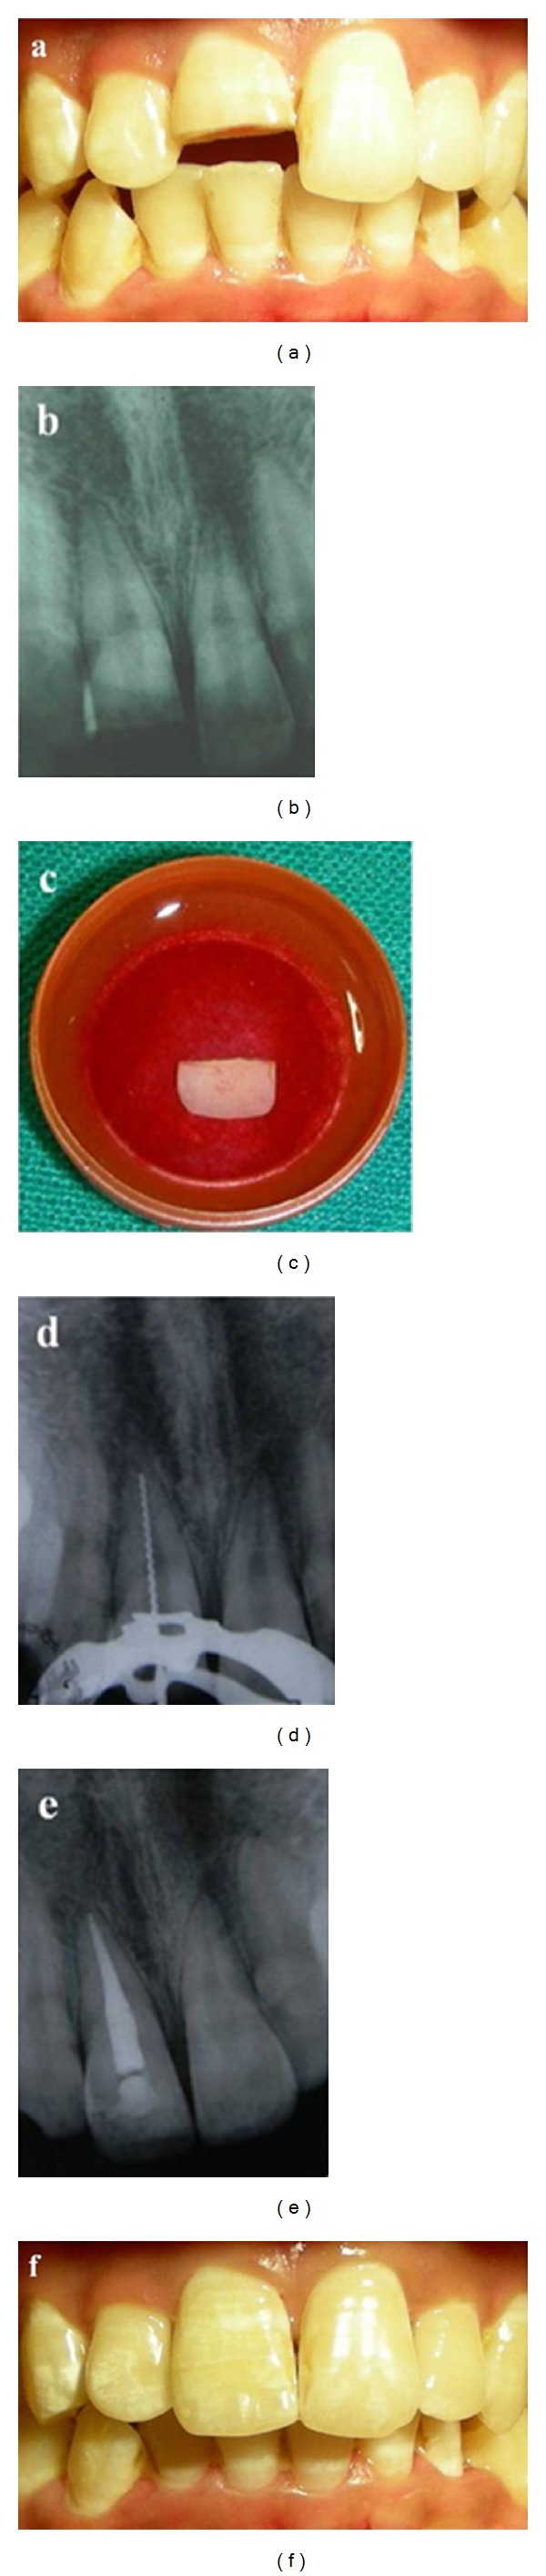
Reattachment of fractured tooth segment. (a) Clinical appearance of the patient, (b) preoperative radiograph, (c) fractured crown segment bought by the patient, (d) working length radiograph, (e) postoperative radiograph, and (f) clinical view after segment reattachment.
